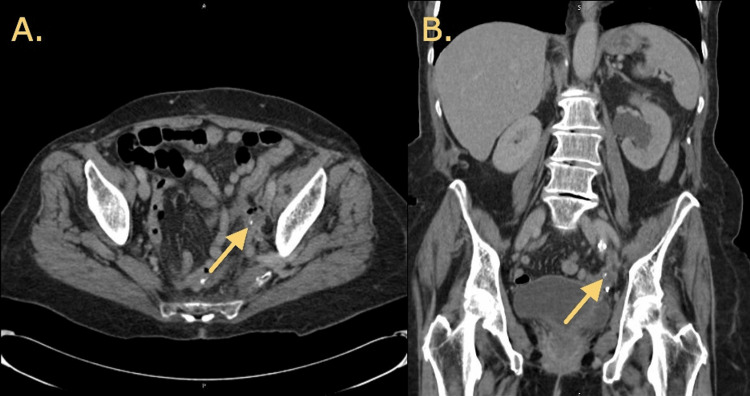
CT imaging demonstrating findings interpreted as a 4-mm obstructing stone. (A) Axial view of CT scan results with arrow indicating region interpreted as a 4-mm stone. (B) Coronal view of CT scan results with arrow indicating region interpreted as a 4-mm stone.
